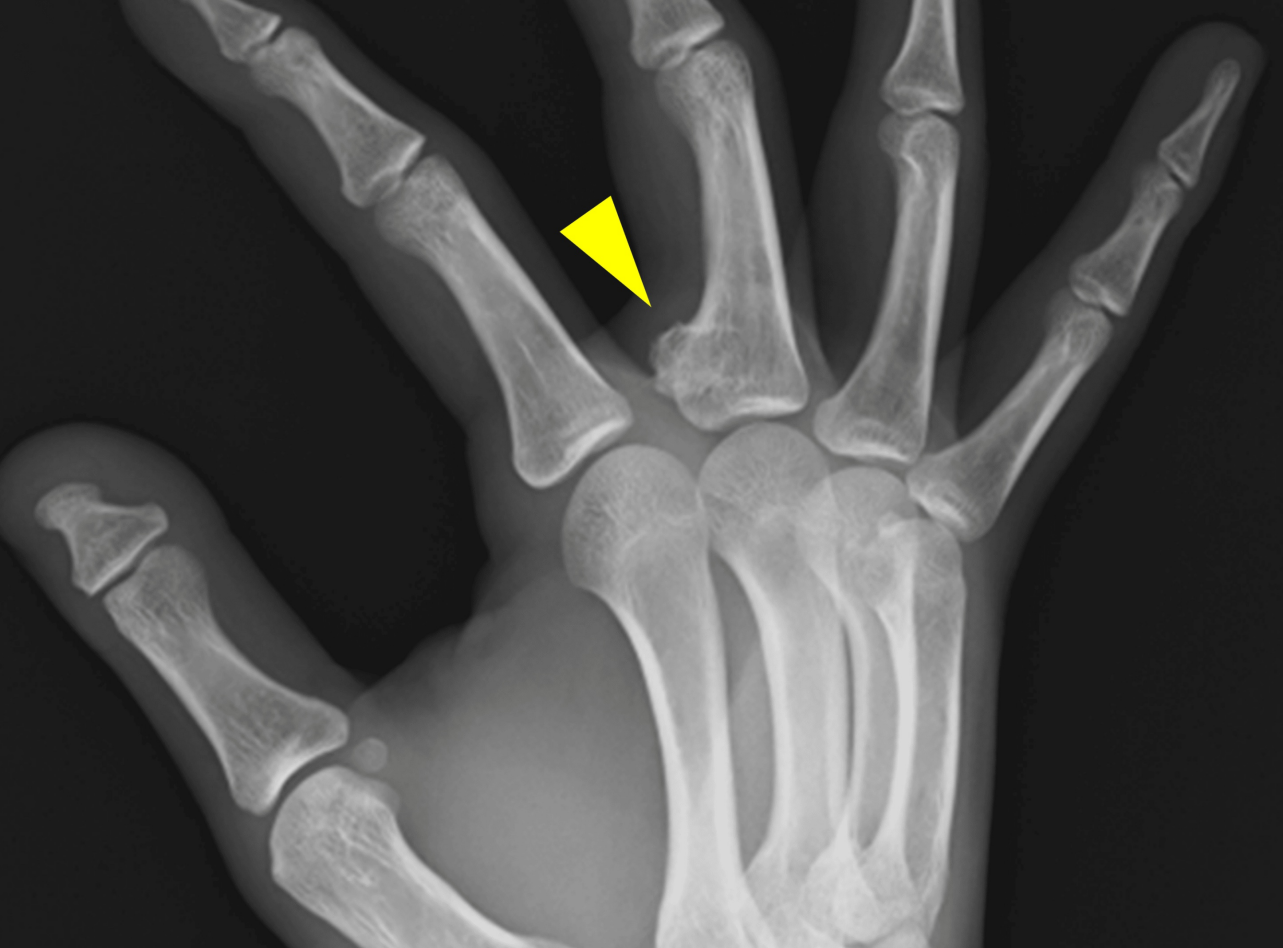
Plain radiograph. A bony prominence on the palmar side of the proximal end of the middle phalanx is visible.
